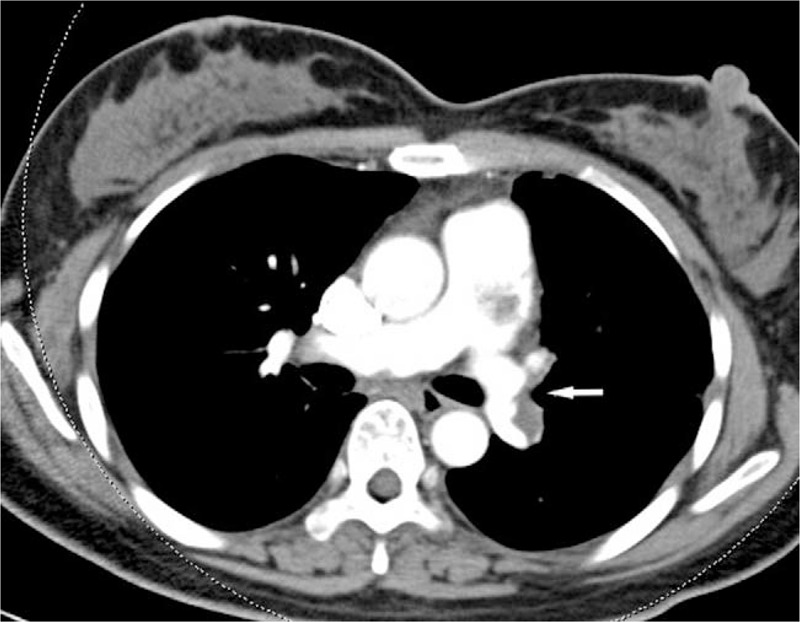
Enhanced chest computed tomography shows an irregular low-density soft tissue lesion (the filling defect is highlighted with white arrow) in the main pulmonary artery and left pulmonary artery.
